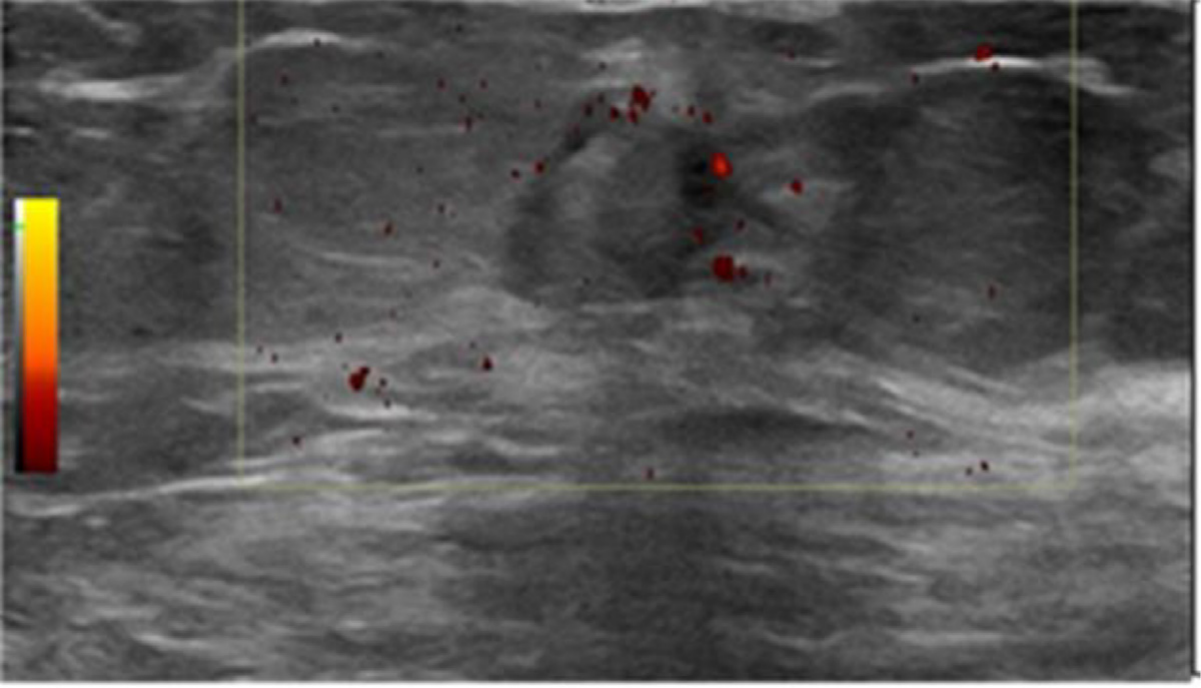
Follow-up ultrasound image after injection confirms pseudo aneurysm thrombosis with no color flow in color doppler study.
